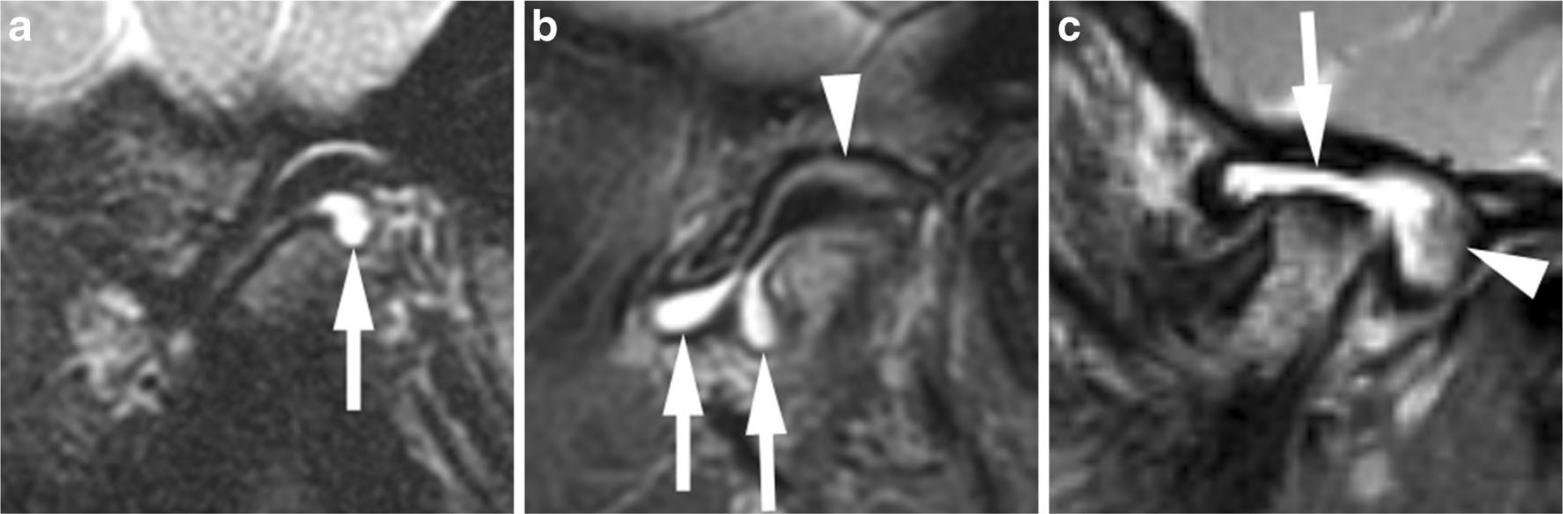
Large joint effusion (grade 2) on sagittal oblique images. a-c Effusion exceeding 2-mm width (arrows) on fat-saturated T2-weighted fast spin echo images (TR/TE 5,400/77 ms) in the posterior recess in a 15-year-old girl (a), in the anterior recesses in a 13-year-old boy (b) and with bulging of the upper joint compartment in a 7-year-old girl (c). Note thickened synovium with intermediate signal intensity (arrowheads) on these posteriorly in (b) and (c)
